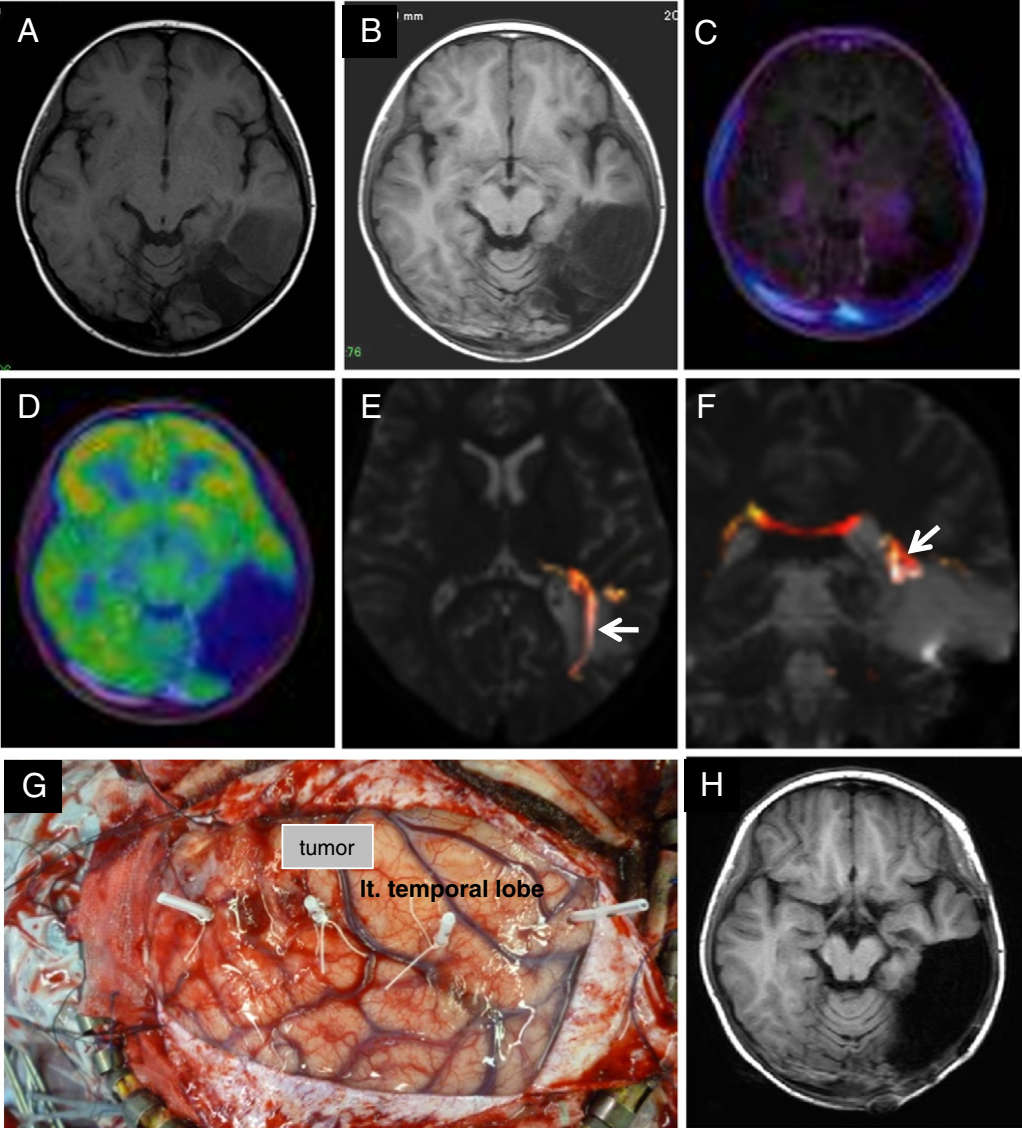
Case 3. T1WI after the first surgery showing a partially resected, well-demarcated, hypointensity mass lesion in the left posterior temporal lobe (A). Two years later, T1WI showing tumor progression (B). The tumor showed low uptake in MET-PET (fused with T1WI) (C) and hypo-uptake in FDG-PET (fused with T1WI) (D). Fiber tractography showed the left visual tract (arrow) to be mediorostral to the tumor (E and F). This is a photograph showing the neuronavigation-guided fence-post tube technique used in the surgery (G). Postoperative T1WI showed that the tumor was totally resected (H).
